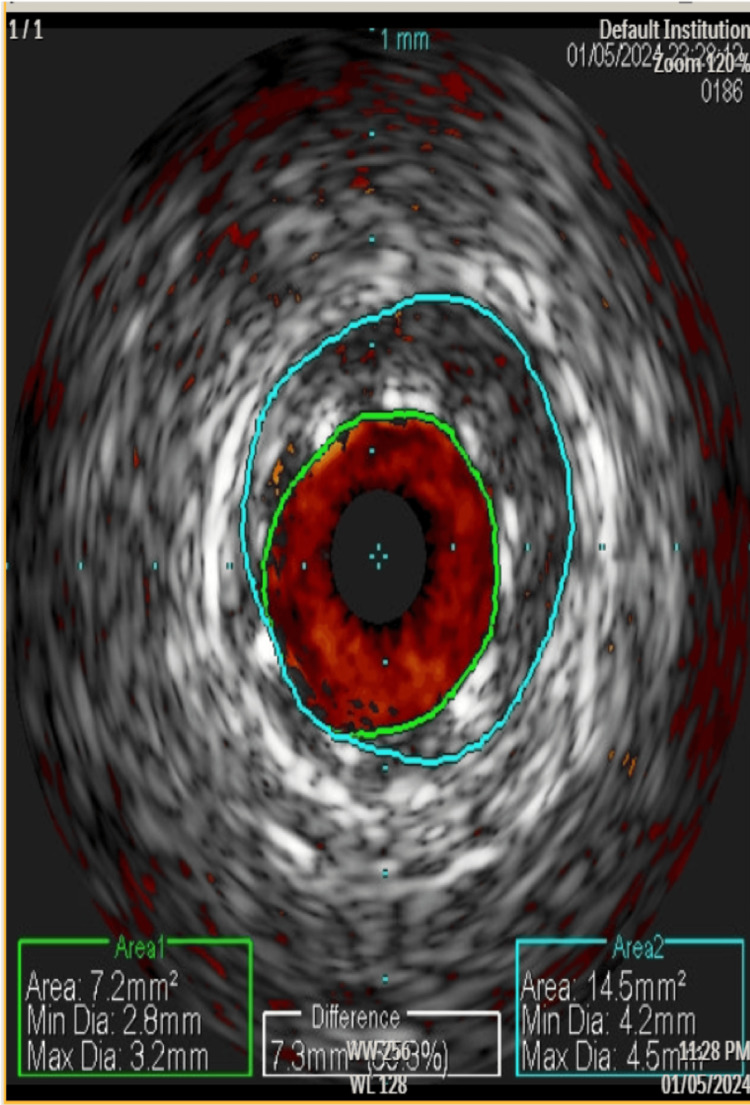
IVUS image post stenting showing possible dissection flap IVUS: Intravascular ultrasound
